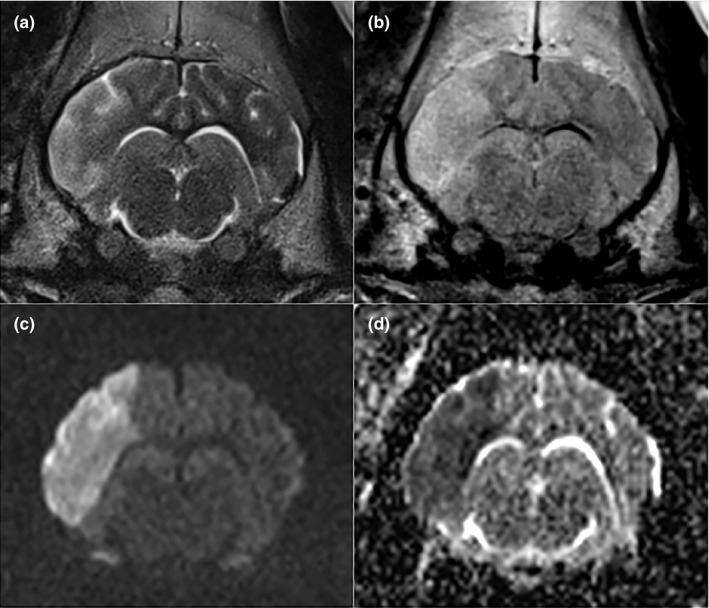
MRI performed 24 hr following MCAO demonstrates ischemic stroke in the territory of the middle cerebral artery. The affected area is hyperintense on T2‐weighted imaging (a) and T2 FLAIR (b) relative to normal grey matter. The region is hyperintense on DWI (c) with corresponding hypointensity on the ADC map (d) confirming cytotoxic edema
